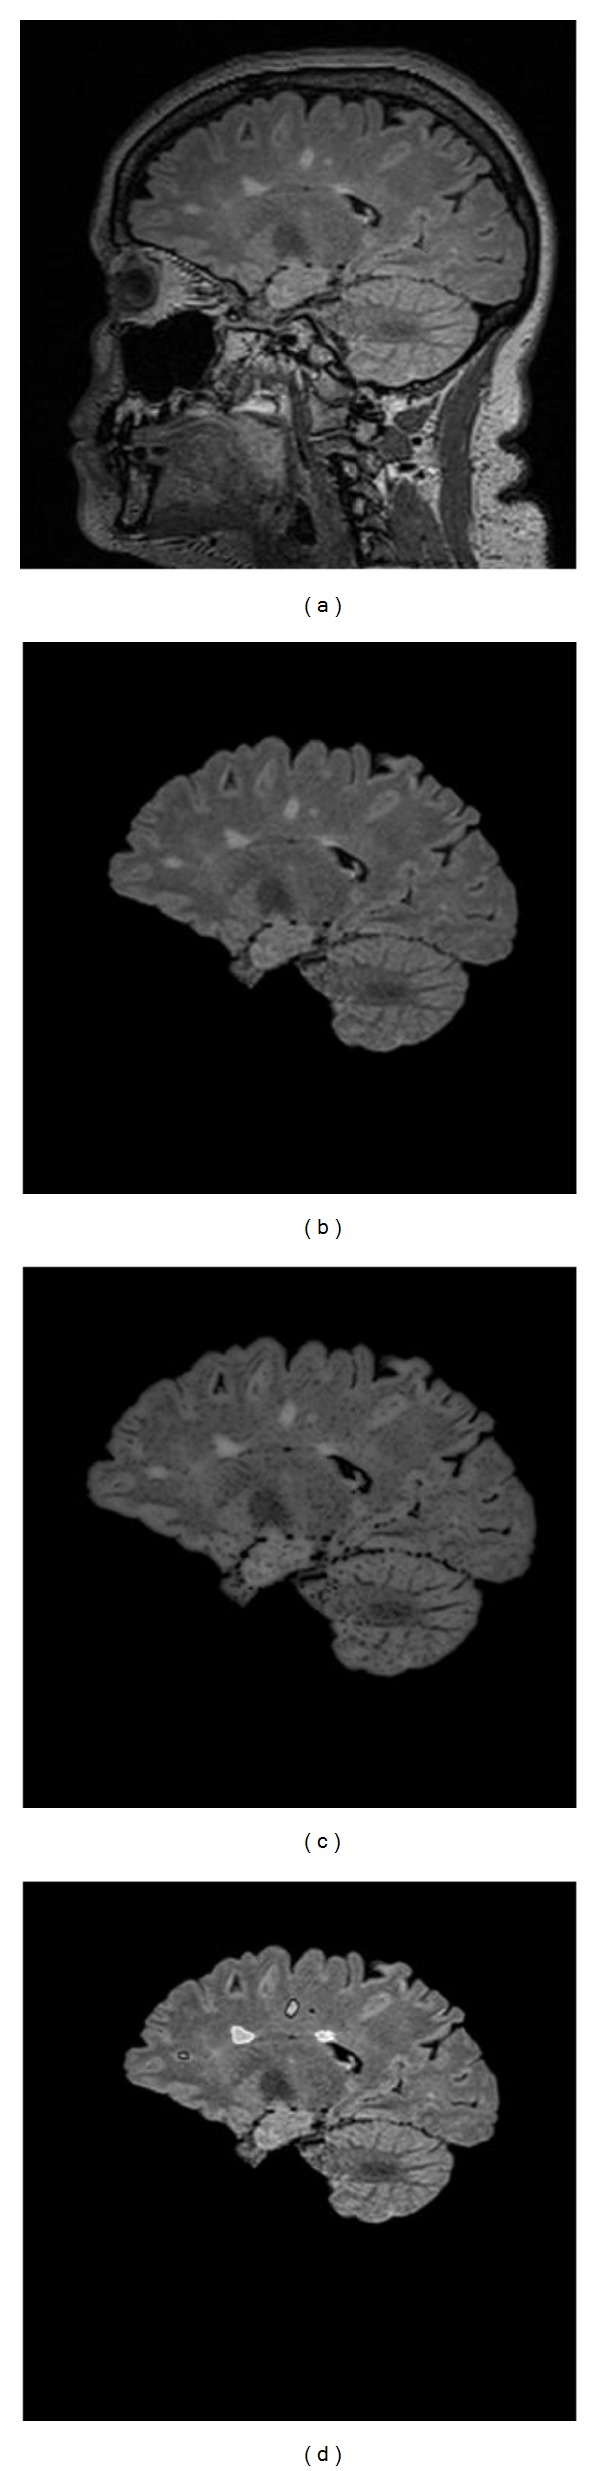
A 3D sagittal view FLAIR brain image. (a) Original images; (b) after skull stripping and bias field correction; (c) after high pass suppression of GM edges; and (d) the final segmentation of lesions and assignment of their locations (black contour for DWMH, white contour for PVH).
